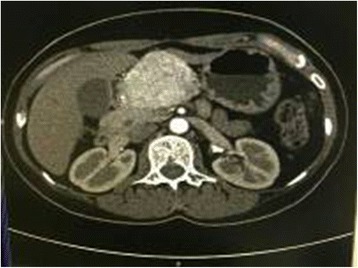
On contrast-enhanced CT, the tumor demonstrated marked enhancement in the arterial phase
